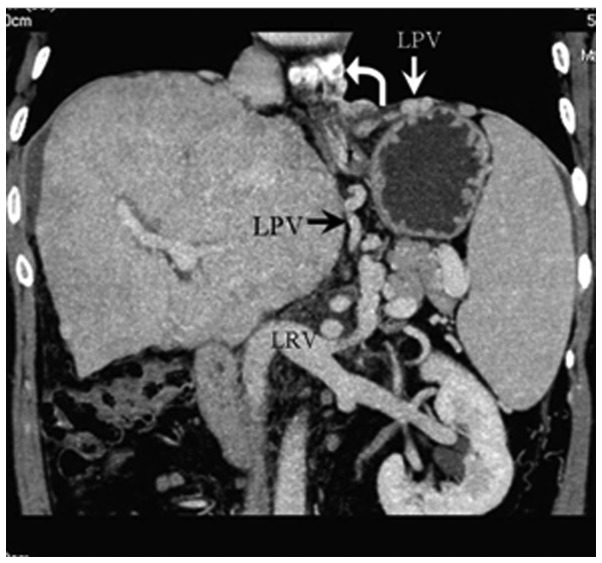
IVC-left renal vein-LPV circulation. Computed tomography angiography shows the dilated left renal vein communicating with the LPV. Tortuous blood vessels in the cardiophrenic angle can also be observed (curved arrow). IVC, inferior vena cava; LPV, left inferior phrenic vein.
